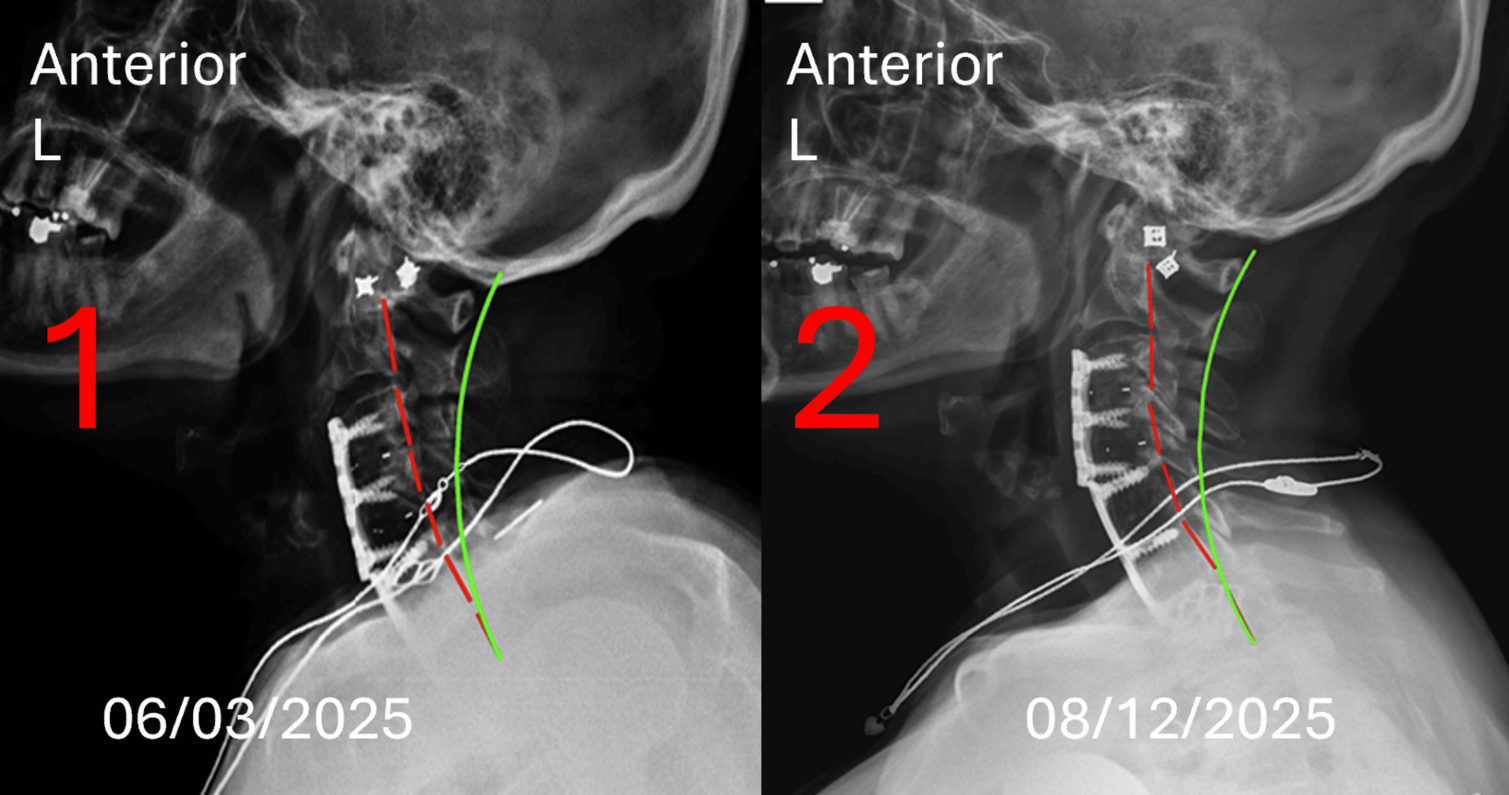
Lateral cervical radiograph compassion The green line depicts the expected alignment of the posterior longitudinal ligament in a normal spine. The red line represents the subjects' alignment as defined by George’s line, reflecting deviation of the posterior longitudinal ligament. The red lines represent the actual posterior tangent lines of the C2-T1 vertebrae using the Harrison posterior tangent method on the lateral cervical radiograph. The green lines represent the ideal spine model. (1) X-ray image represents the initial presentation to the office on 06/03/2025. Pre-treatment neutral lateral cervical radiograph with a cervical curvature ARA C2-C7 measuring -20.4° (ideal measured at -42°, normal is -34°, and pain threshold is -20°) and anterior head translation C2-C7 measuring 21.4 mm (ideal measured at 0 mm, normal is 10 mm, and pain threshold is 20 mm). (2) X-ray image represents the day of discharge from the office on 08/12/2025. Post-treatment neutral lateral cervical radiograph with ARA C2-C7 measuring -23.2° and C2-C7 measuring 19.9 mm. The Absolute Rotation Angle (RRA) tool is a radiographic measurement method used to assess the total angle of spinal curvature.
